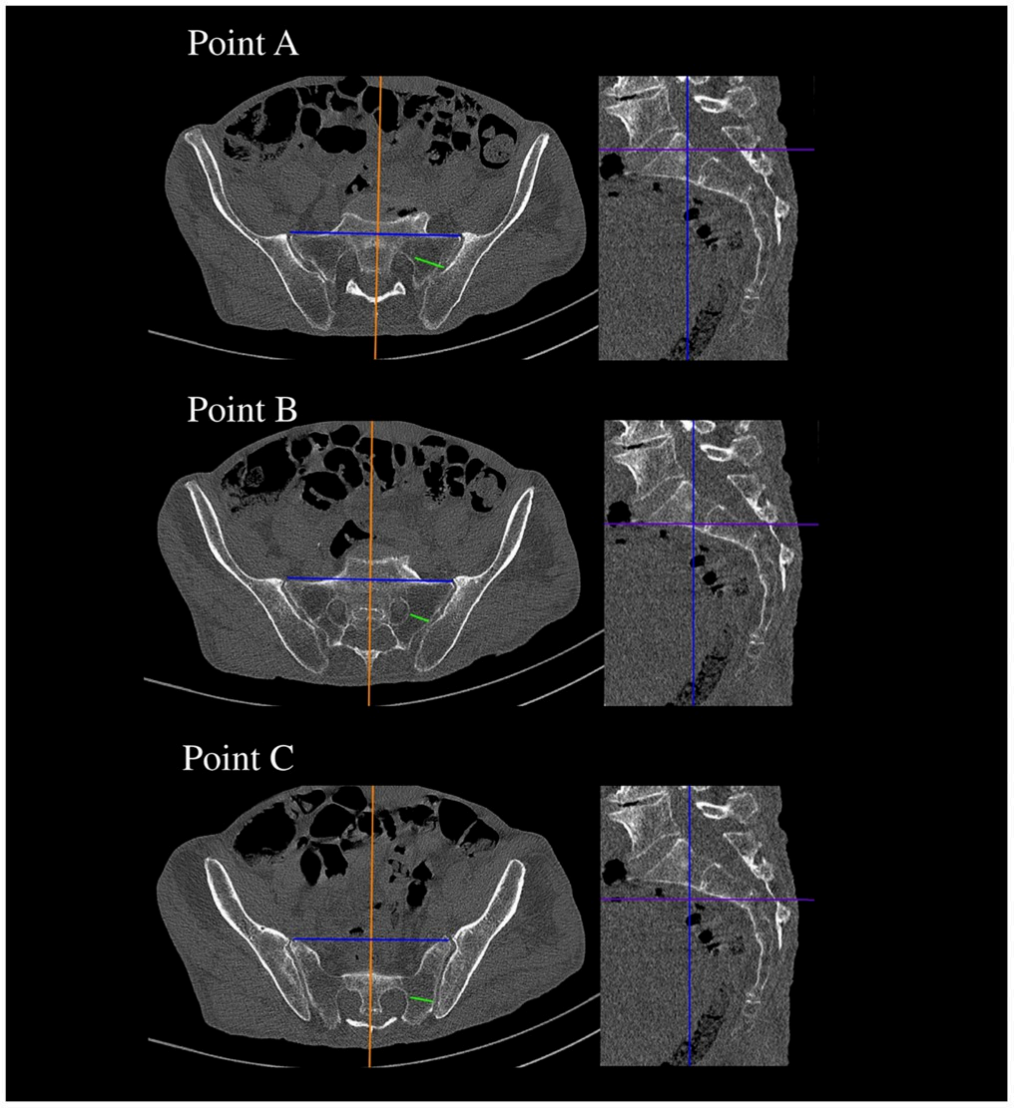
CT showing the widths between SIJ and the sacral canal that correspond to safe corridors for screws at points A, B, and C.
